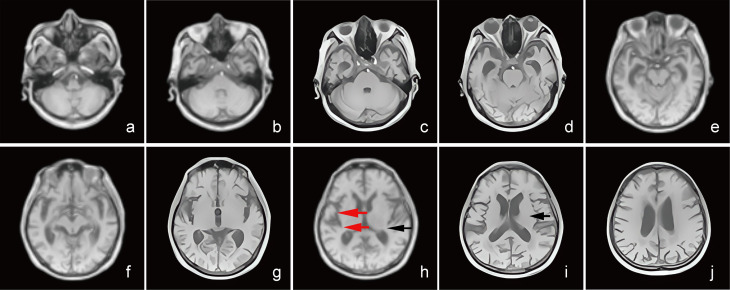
Brain MRI T1WI sequence imaging of AD patients. (a–j) The T1WI
